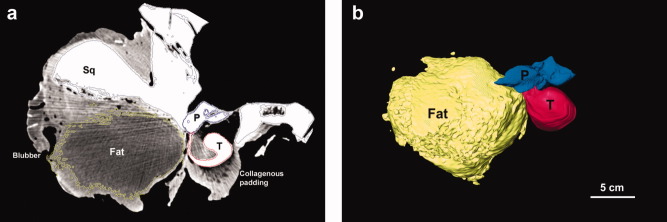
Posterior view of the partially dissected left ear region of B-acu19. (a) Axial CT image showing the ear fat extending from the blubber region to the tympanoperiotic complex (ears). Most of the blubber has been trimmed, but the remaining parts can be seen on the far left side of the image. The collagenous padding is covering the ventral portion of the tympanic bone. (b) Three-dimensional reconstruction. Yellow, ear fats; blue, periotic; red, tympanic. Abbreviations: T, tympanic; P, periotic; Sq, squamosal.
